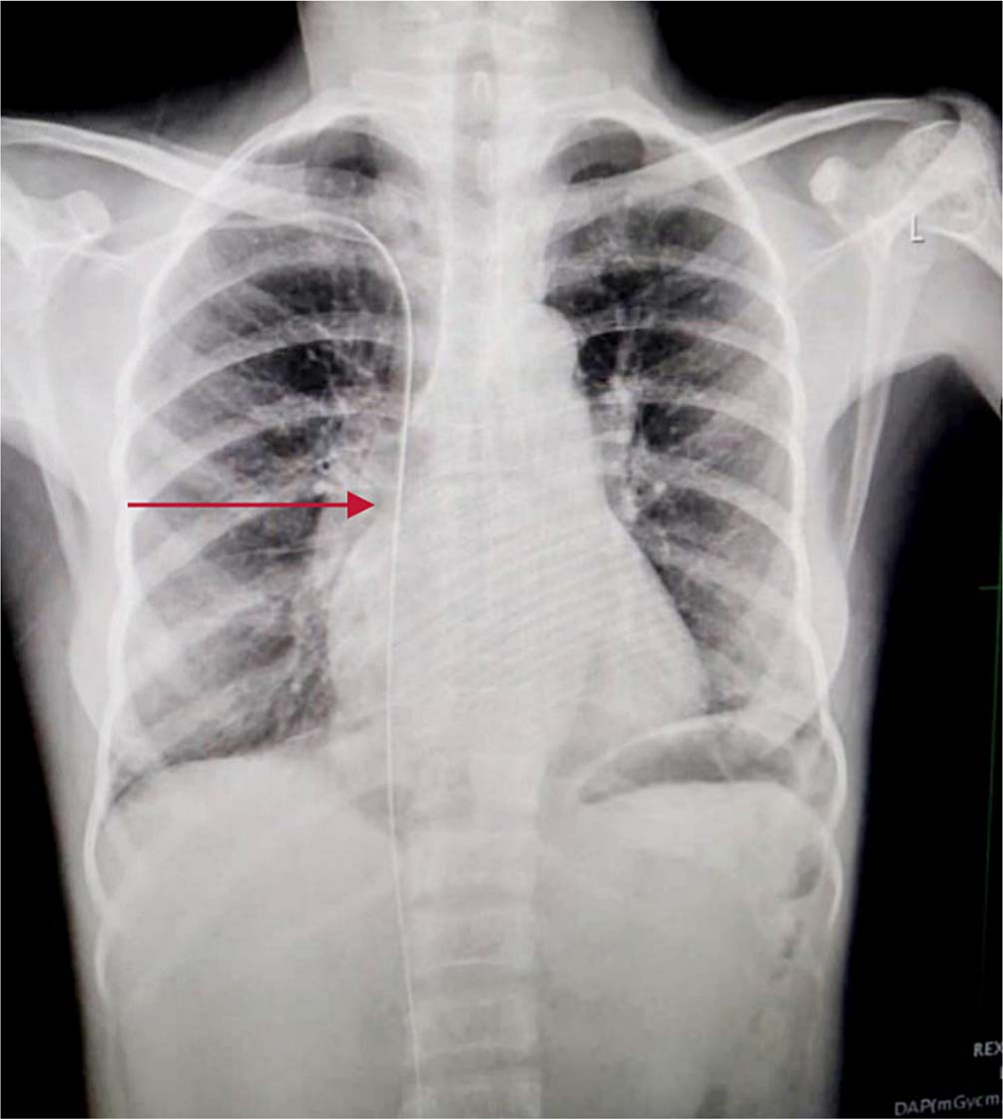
Chest X-ray showing guide wire extending from the right subclavian vein to the SVC and via the right atrium to the IVC.
